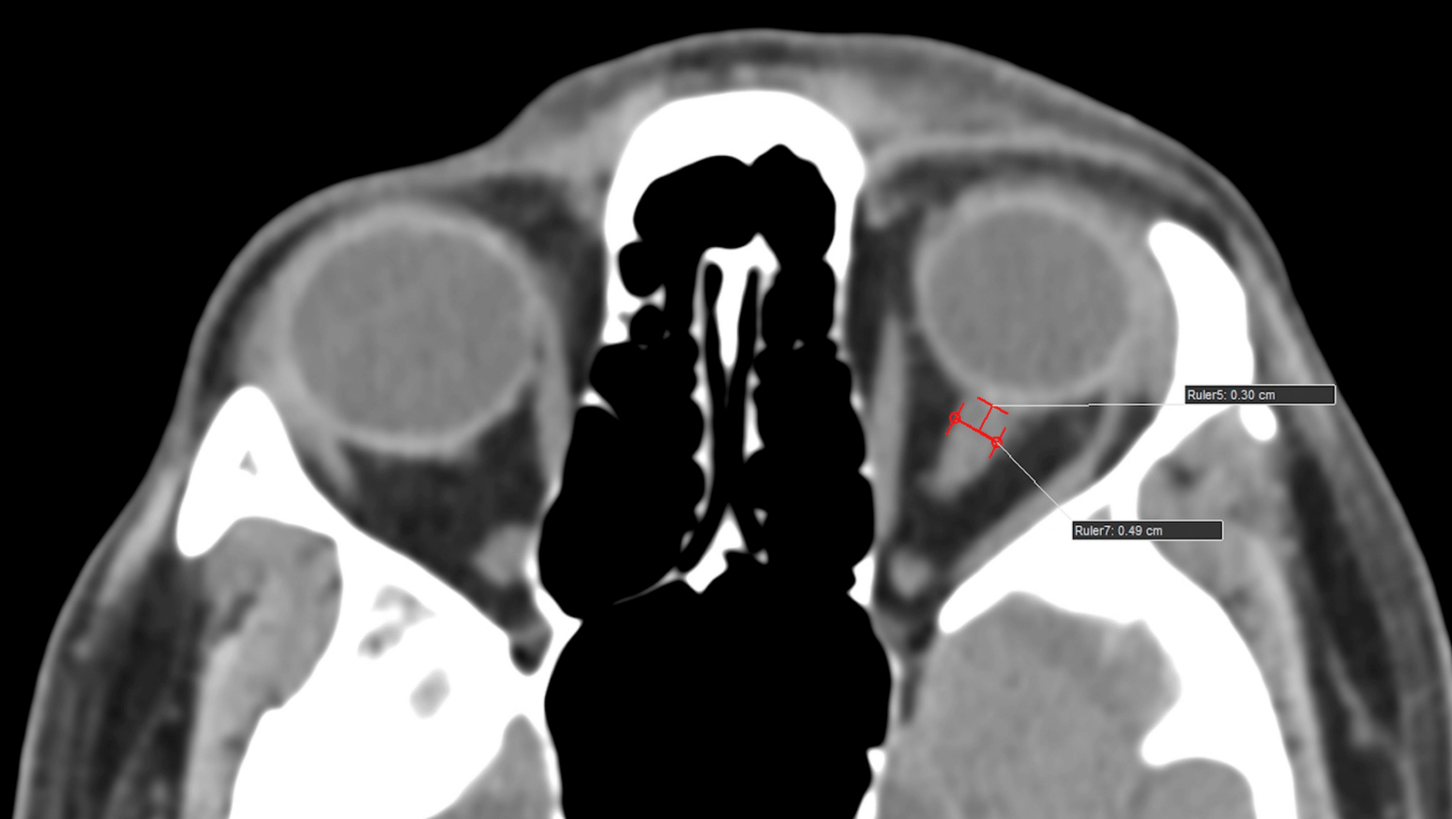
An average healthy subject/individual. A computed tomography (CT) scan of the brain (axial section, 1 mm slice thickness, standard mediastinum algorithm, window level of 10 Hounsfield units {HU} and width of 300 HU) shows optic nerve sheath diameter (ONSD) measured transversely (3 mm behind the globe).
